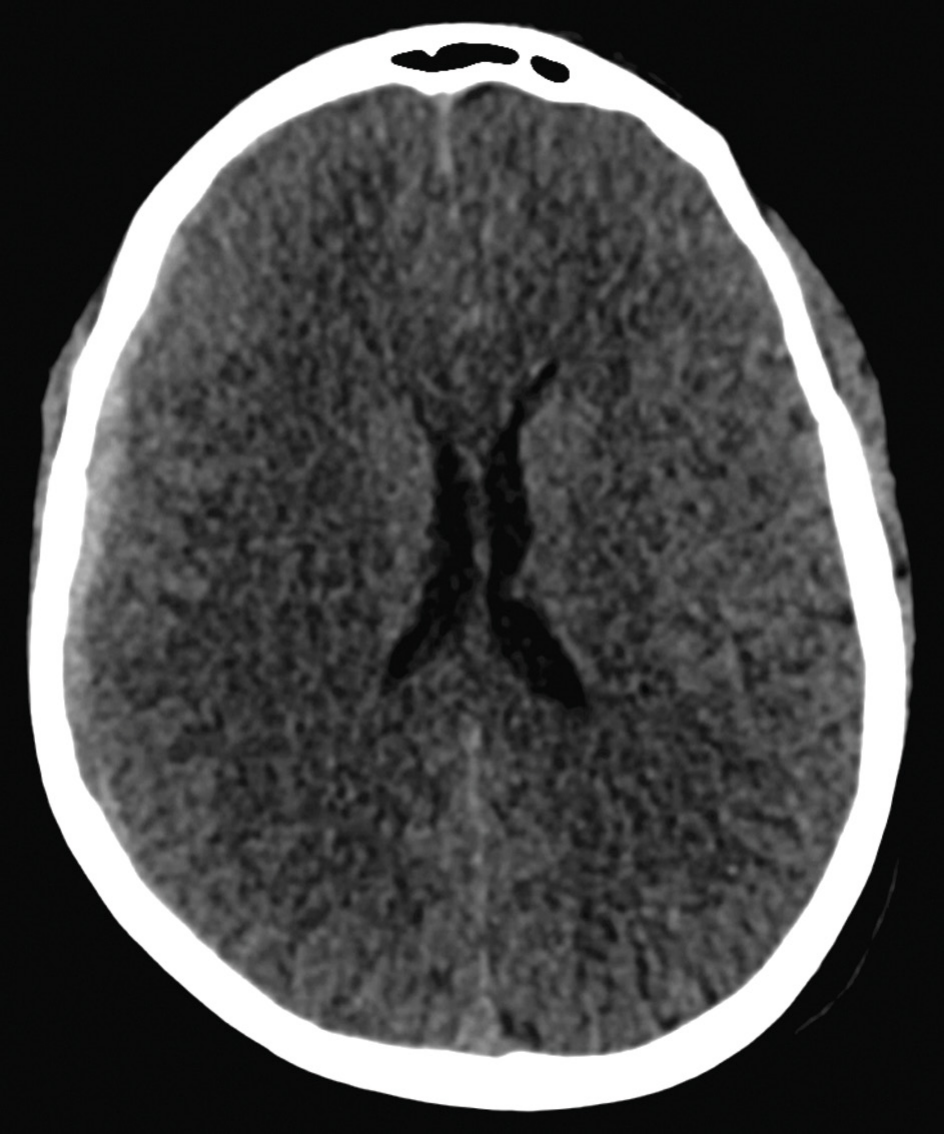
Non‑enhanced CT of the brain revealing a right hemispheric subdural hematoma.
